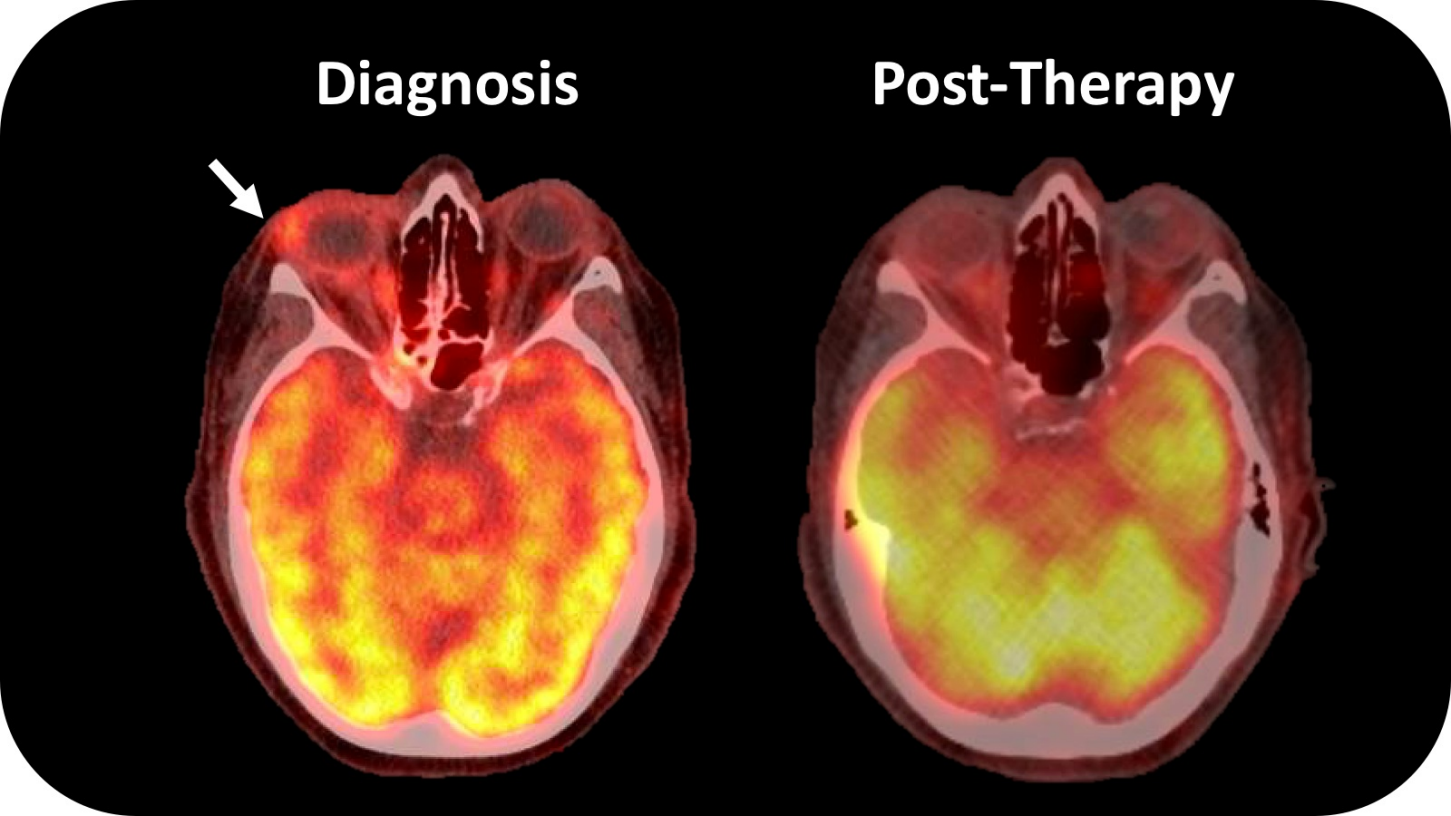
Positron emission tomography–computed tomography of the orbits at diagnosis and post-treatment Depicts PET/CT of the orbits at diagnosis and 11 months after anastrozole and proton radiotherapy. The PET/CT at diagnosis demonstrates diffuse abnormal uptake with focal areas of high standardized uptake (white arrow). After treatment, the generalized uptake is decreased and the focal areas are resolved.
